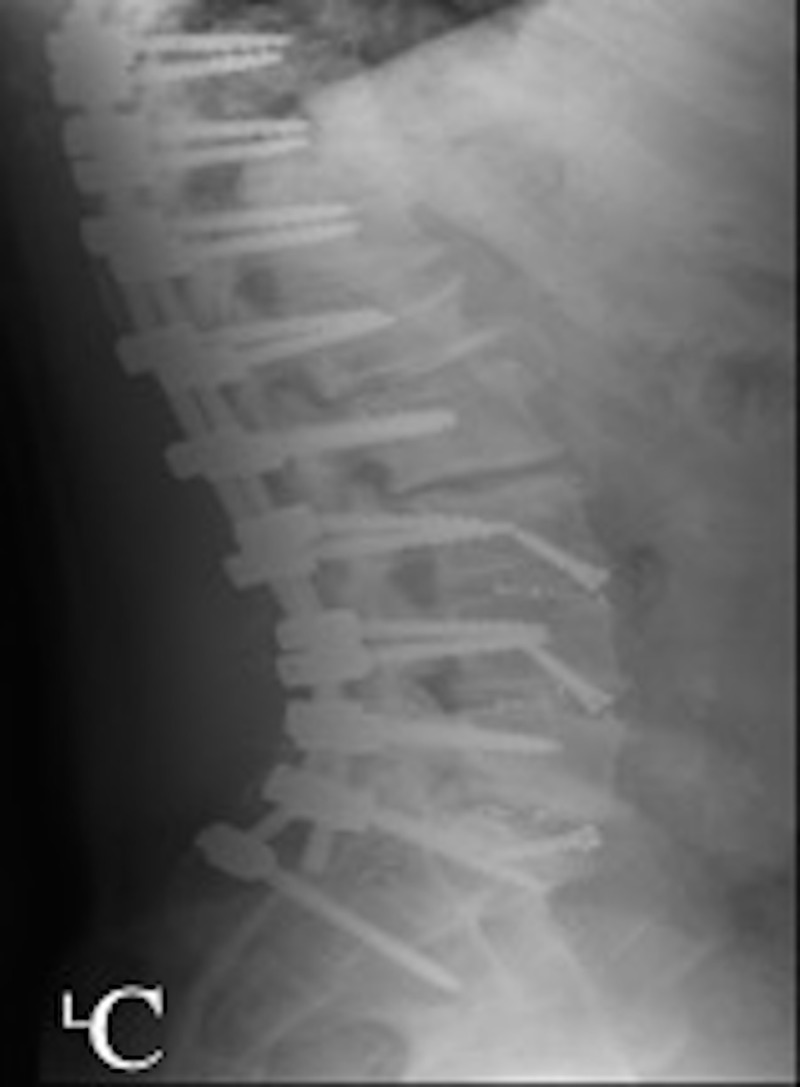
Post-operative lateral X-rays. Lateral post-operative X-ray demonstrating anterior interbody fusion cages at L3-S1. Posterior instrumentation from T10 to the pelvis is also seen.
